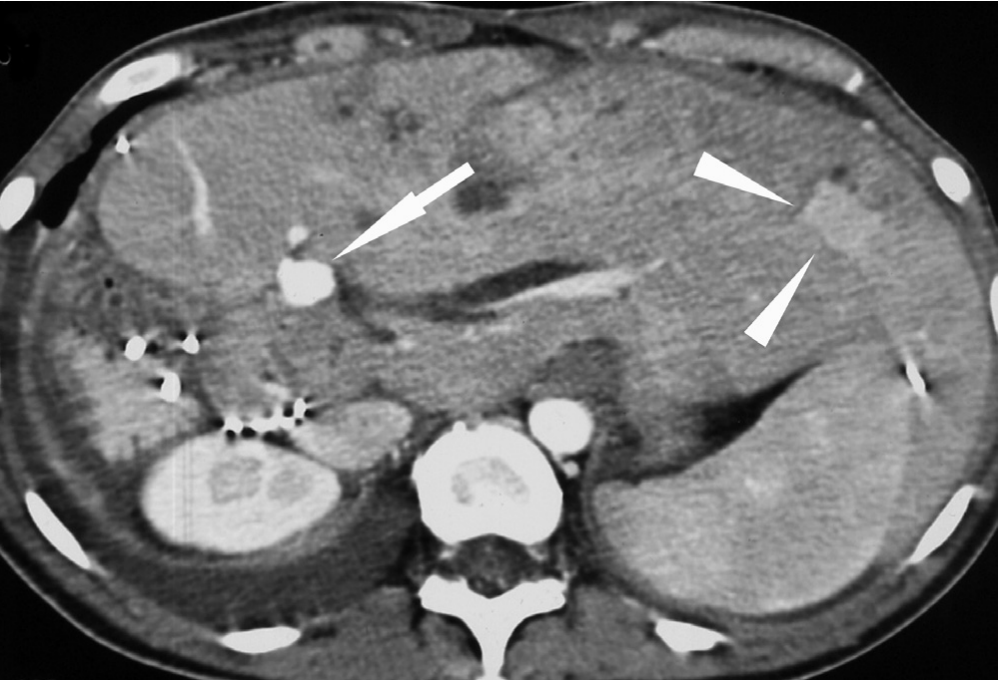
Contrast-enhanced abdominal CT. The left liver lobe is hypertrophied after extended right hemihepatectomy. There is an arterial pseudoaneurysm *(arrow) *close to the hepato-enteric anastomosis. A hypervascular metastasis is depicted on the same cut *(arrowheads)*.
